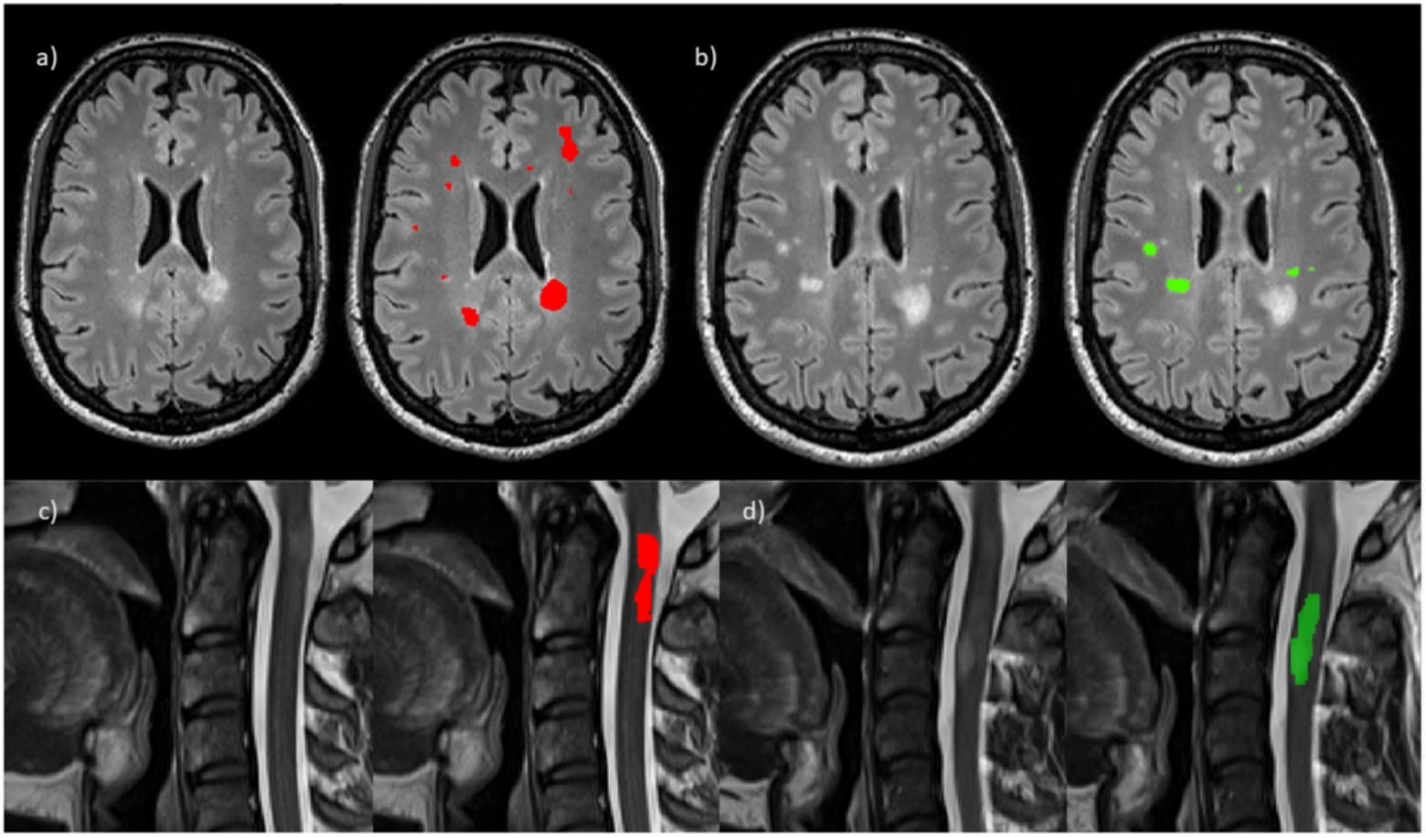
Examples of brain and spinal cord lesions segmentation at baseline and follow up (M12) in the same patient. a Baseline brain lesions segmentation on 3D FLAIR using a deep-learning based tool (red mask); b new brain lesion segmentation at follow-up identified with the help of a deep-learning based tool and registration (green mask); c baseline spinal cord lesions segmentation on sagittal T2 using a deep-learning based tool (red mask); d manual segmentation of a new spinal cord lesion segmentation at follow-up (green mask)
